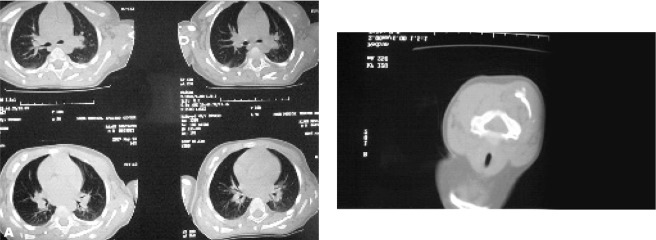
A. Thorax and B. Neck CT scan indicates soft tissue ossification in cervical and thoracic paravertebral soft tissue masses in the same case one year later at two years of age
